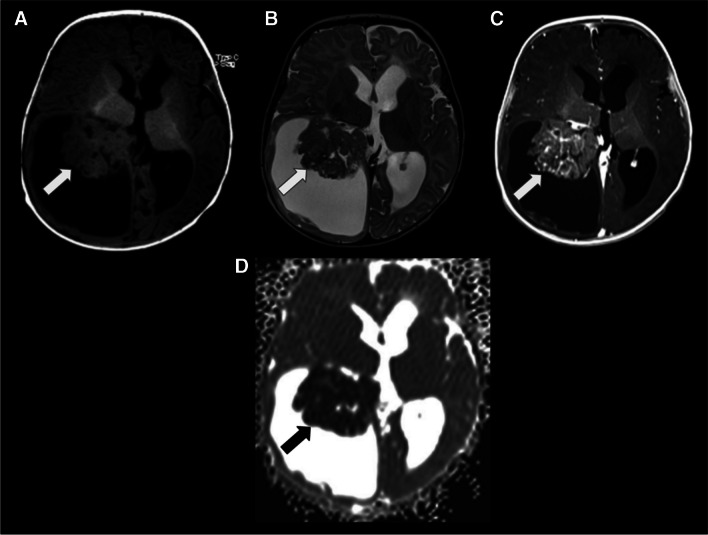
Intraventricular ATRT (whiter arrows). A The axial T1-weighted image displays an irregular, isointense, lobulated intraventricular mass associated with hydrocephalus. B The axial T2-weighted image reveals the mass to be diffusely hypointense, with central cystic/necrotic degeneration. Notably, the lesion is partially attached to the ventricular wall and associated with a marked enlargement of the right lateral occipital horn, likely entrapped. C The axial contrast-enhanced T1-weighted image indicates that the lesion has moderate and heterogeneous enhancement. D The axial apparent diffusion coefficient (ADC) map image demonstrates that the lesion exhibits marked restricted diffusion
